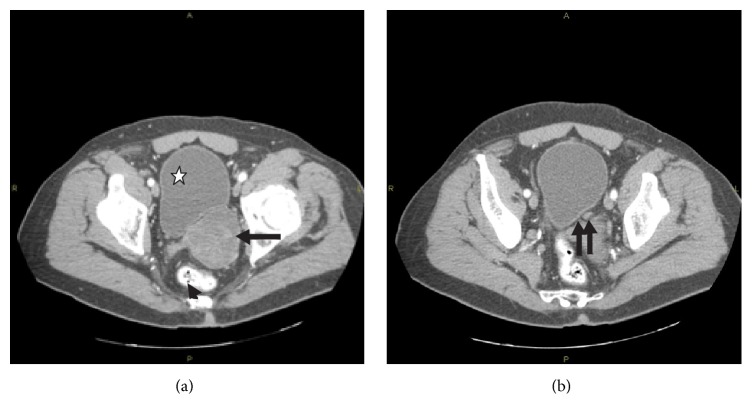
Abdominal/pelvic CT scan image demonstrating (a) a solid, lobed, well-circumscribed mass (arrow) compressing the rectum (arrowhead) and the posterior wall of the urinary bladder (white star) and (b) the distal part of the left ureter (double arrow) between the mass and the bladder wall.
